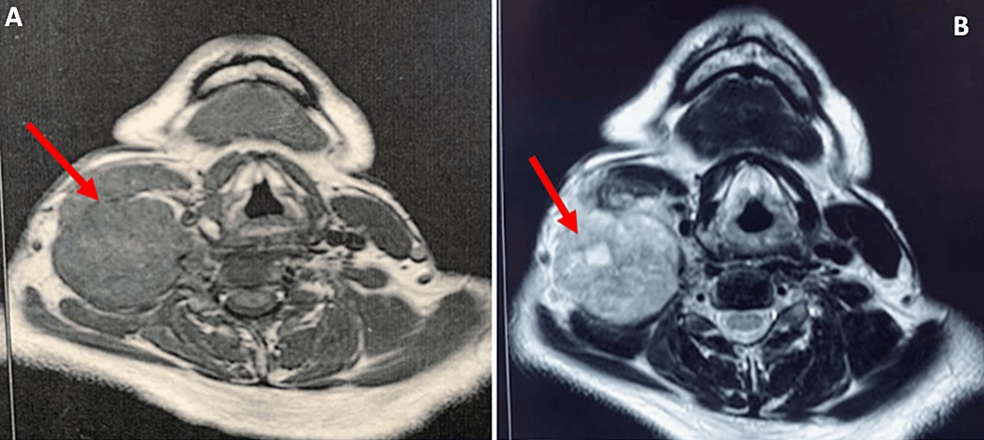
Axial T1-weighted (A) and T2-weighted (B) MRI of the nasopharynx. A cervical nodular mass in the right region (red arrows) that includes areas of necrosis and extensive invasion of the surrounding fat.
